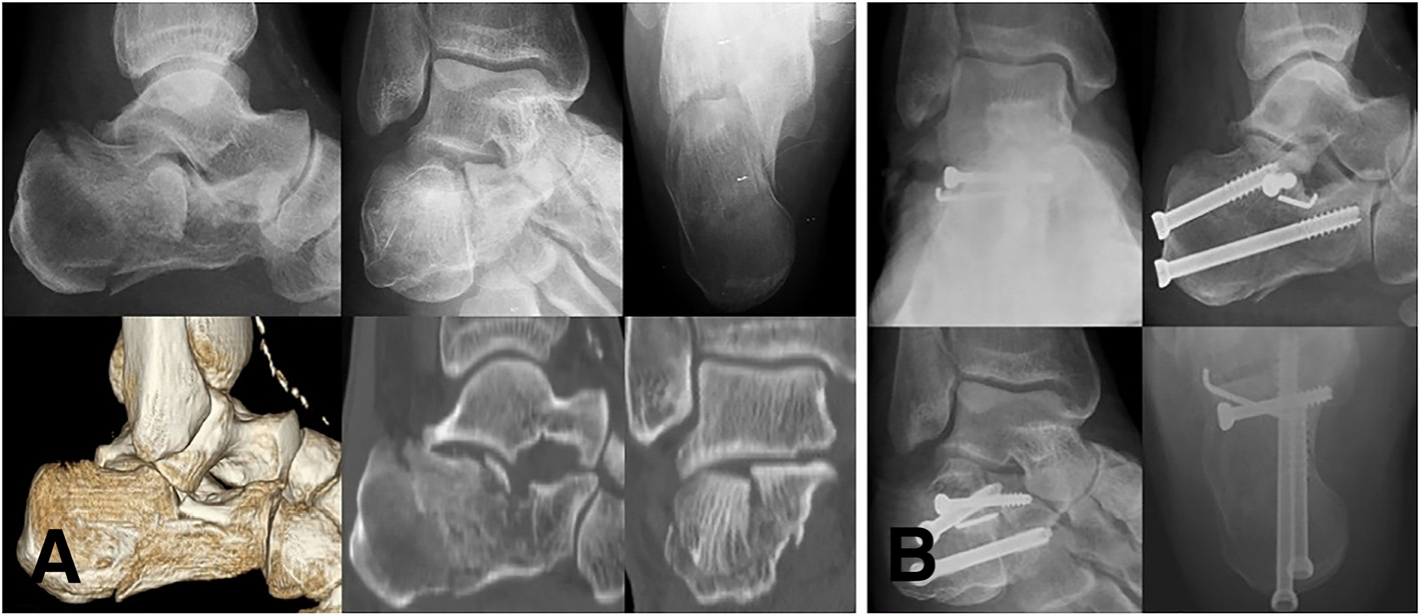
Radiologic evaluations of a 55 year old male patient with Sanders type-II intra-articular calcaneal fracture treated with open reduction and internal fixation using the sinus tarsi approach. (A) Preoperative plain radiographs and CT scans (B) Postoperative plain radiographs.
